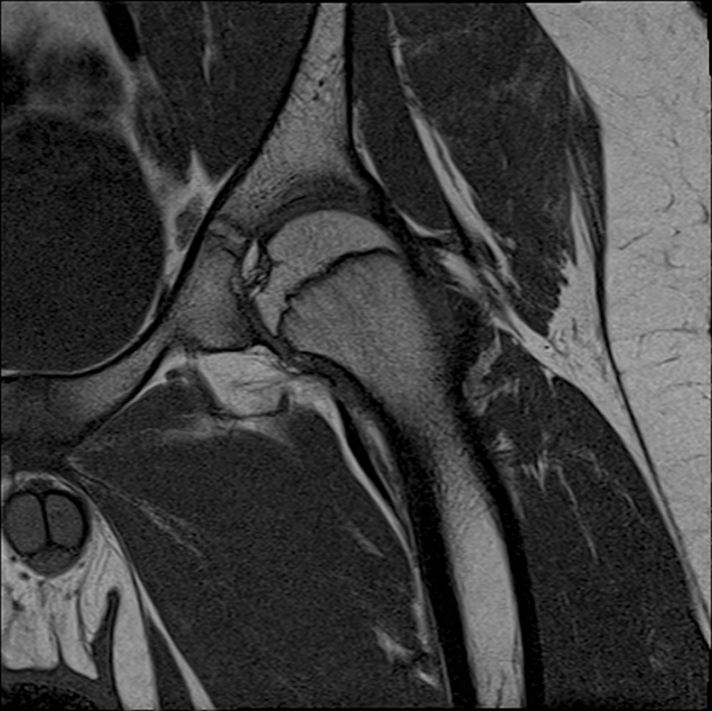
Coronal T1-weighted image of the left hip, showing a single iliopsoas tendon (no bright, fatty cleft separating it in two)
